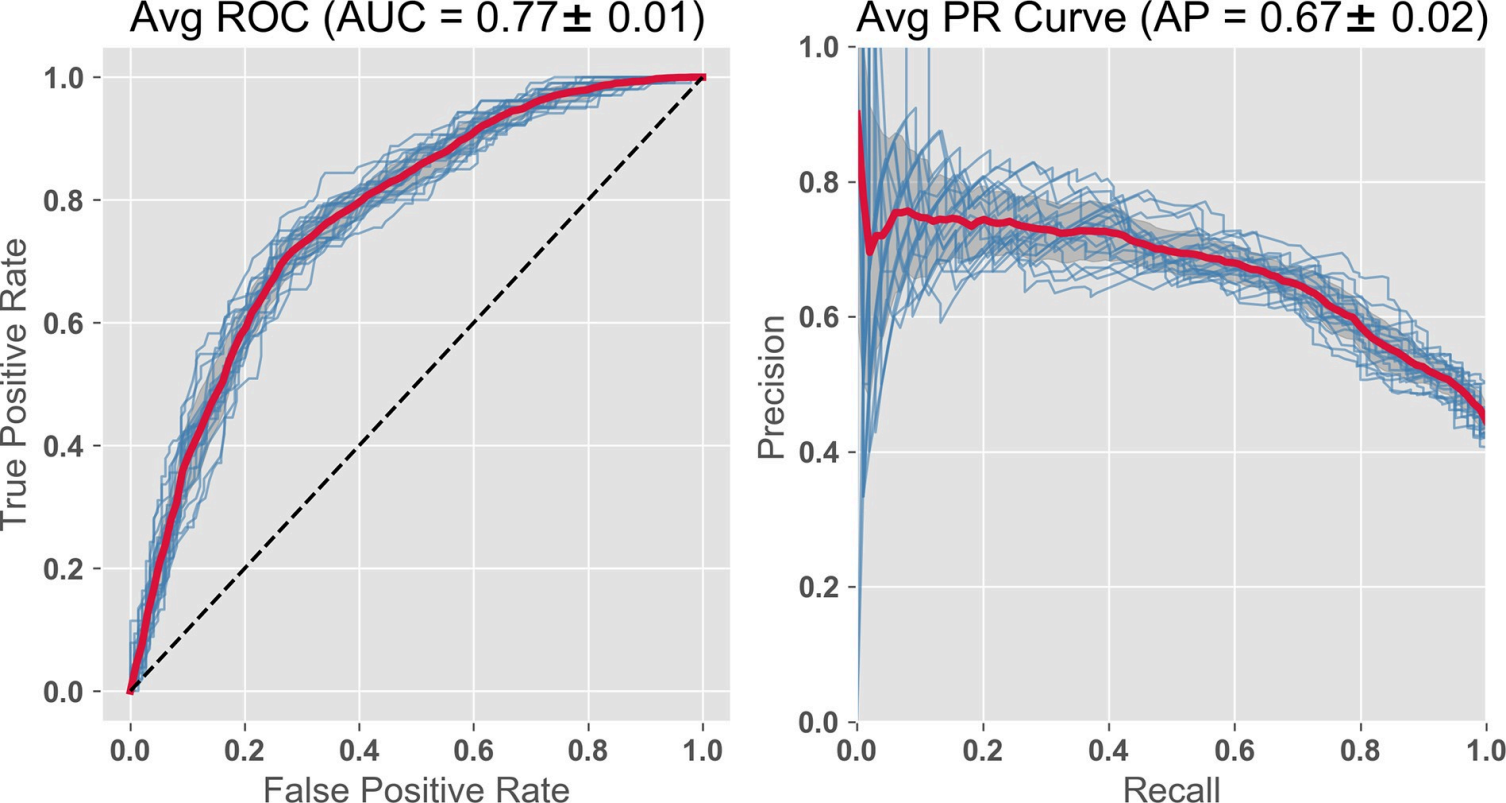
Further AUC ROC improvement is seen combining pBM, MRI, cognitive scores, atrophy rate and demographics. To the features used in Fig 6, we add 19 atrophy features (APC), estimates of annual % change. This improves the AUC from 0.75 to 0.77. The specific MRI regions of interests used to generate atrophy variables are shown in Table 3 where provided is the ADNI code, the neuroanatomical name, and GINI coefficient weighting.
